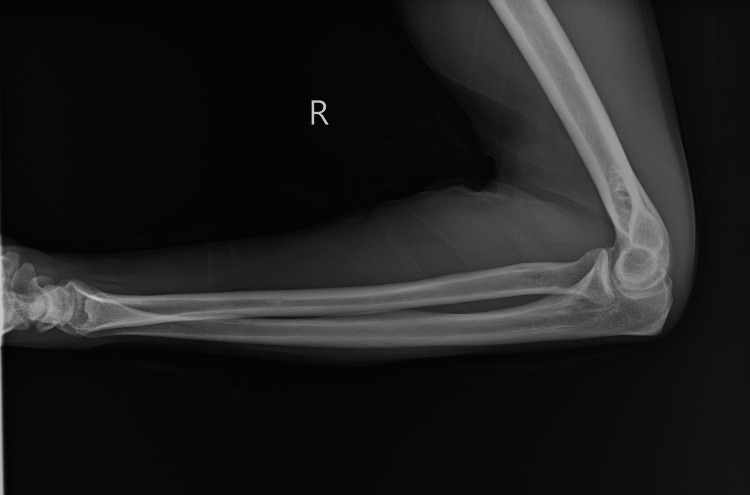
Lateral View Right Forearm with Wrist and Elbow Joints X-ray
